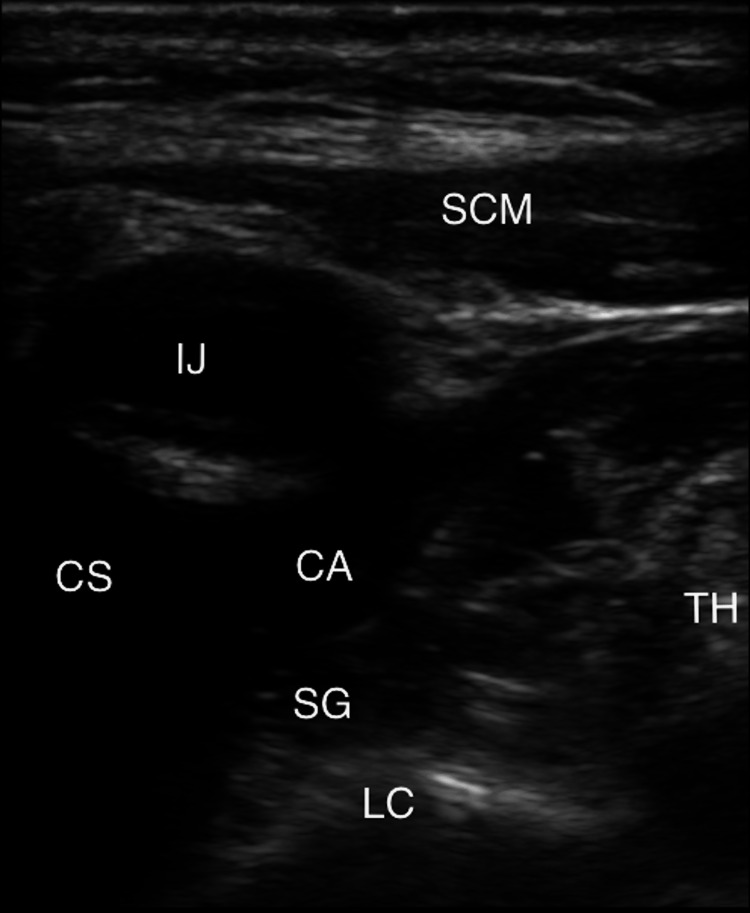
Right stellate ganglion block Ultrasound view of percutaneous blockade of the right stellate ganglion. Bilateral blocks were performed during the resuscitation. The C6 vertebral body likely lies deep (below) the visualized region on this ultrasound image frame. The injection is targeted slightly medial to lateral, beneath the carotid artery, and then lidocaine is injected to visualize the separation of the muscular and fascial layers to confirm correct placement. A bilateral blockade was performed. SCM - sternocleidomastoid muscle, IJ - internal jugular vein, CS - clavicular shadow, CA - carotid artery, SG - stellate ganglion, TH - thyroid tissue, LC - longus colli muscle.
